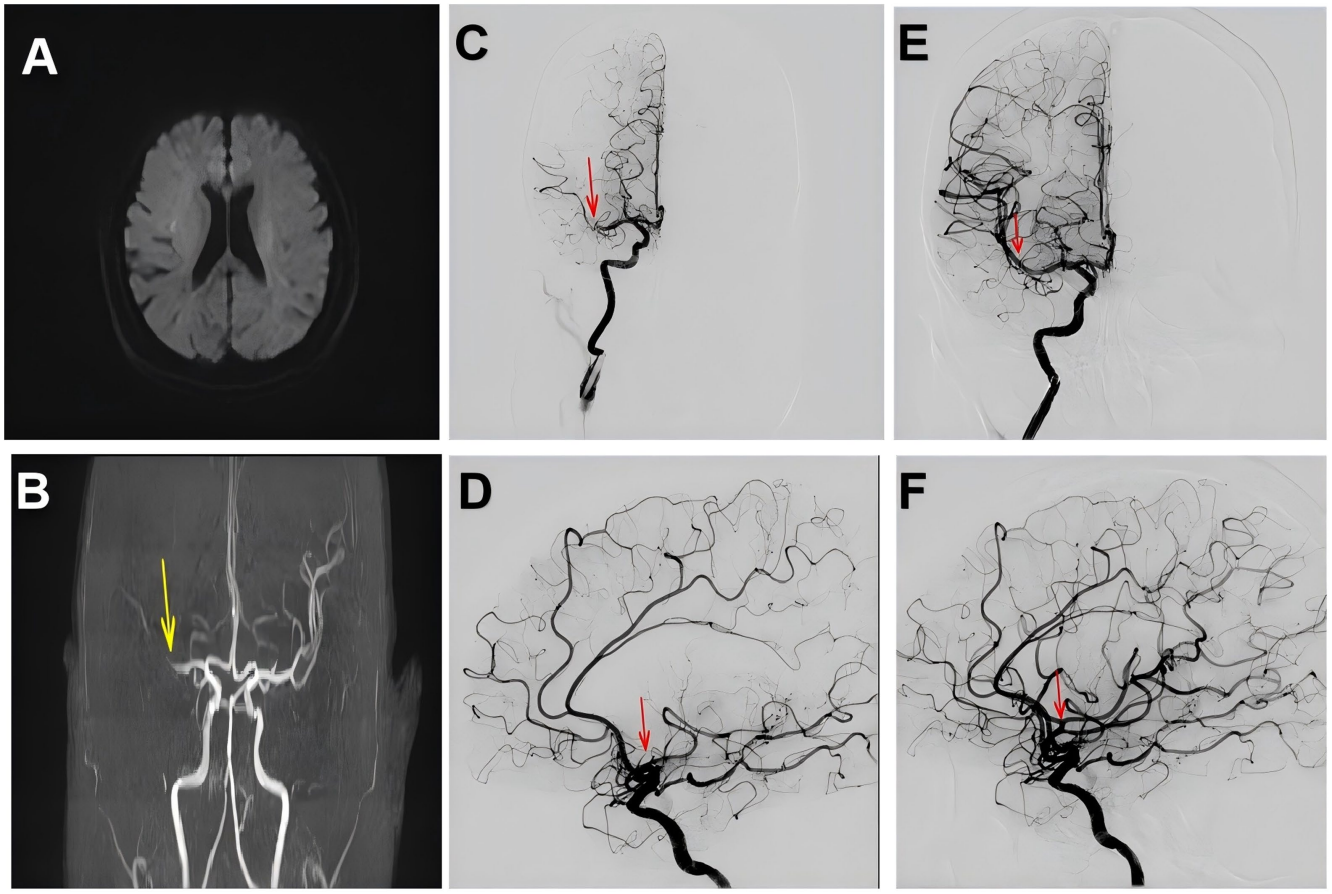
(A) Brain MRI showing multiple punctate abnormal signal foci in the periventricular and centrum semiovale regions bilaterally. (B) Brain MRA demonstrating stenosis of the right middle cerebral artery (M1 segment) indicated by the yellow arrow, with reduced distal branches. (C,D) Right cerebral angiography showing occlusion of the right middle cerebral artery. (E,F) Right cerebral angiography showing restoration of blood flow in the right middle cerebral artery, indicated by the red arrow indicated by the red arrow.
